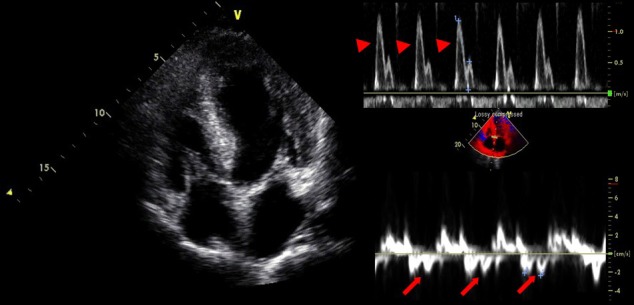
The septal and free walls of the left ventricle were mildly thickened, and the left ventricular cavity dimension was enlarged. Doppler analysis of transmitral inflow showed restrictive pattern with high E/A ratio (2.3) (arrowheads). Tissue Doppler of mitral annular diastolic velocity (E’) was markedly re­duced (2 cm/s) (arrows).
